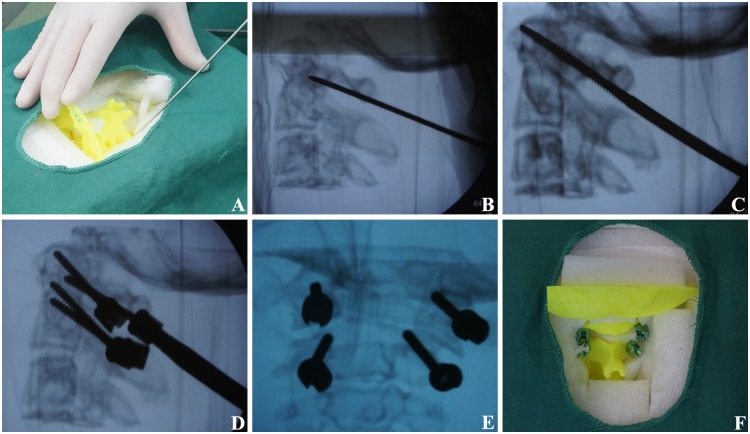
Pedicle screw fixation under C-arm X-ray machine fluoroscopy. (A) The entrance point was determined according to anatomic marker. (B) The direction of long axis of vertebral pedicle were explored under fluoroscopy. (C) The optimal placement channel was explored under fluoroscopy. (D) The entry angle was adjusted under fluoroscopy. (E) Frontal radiograph was adopted after screw fixation; (F) Pedicle screw fixation was finished.
